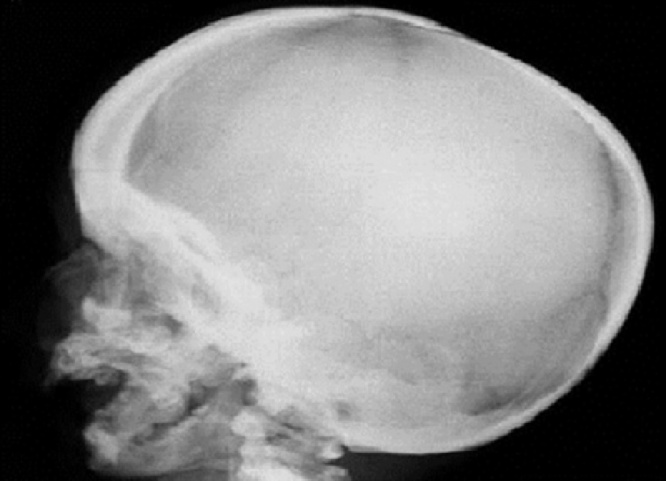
The direct x-ray examination did not show a prominent fracture in the nasal region.
